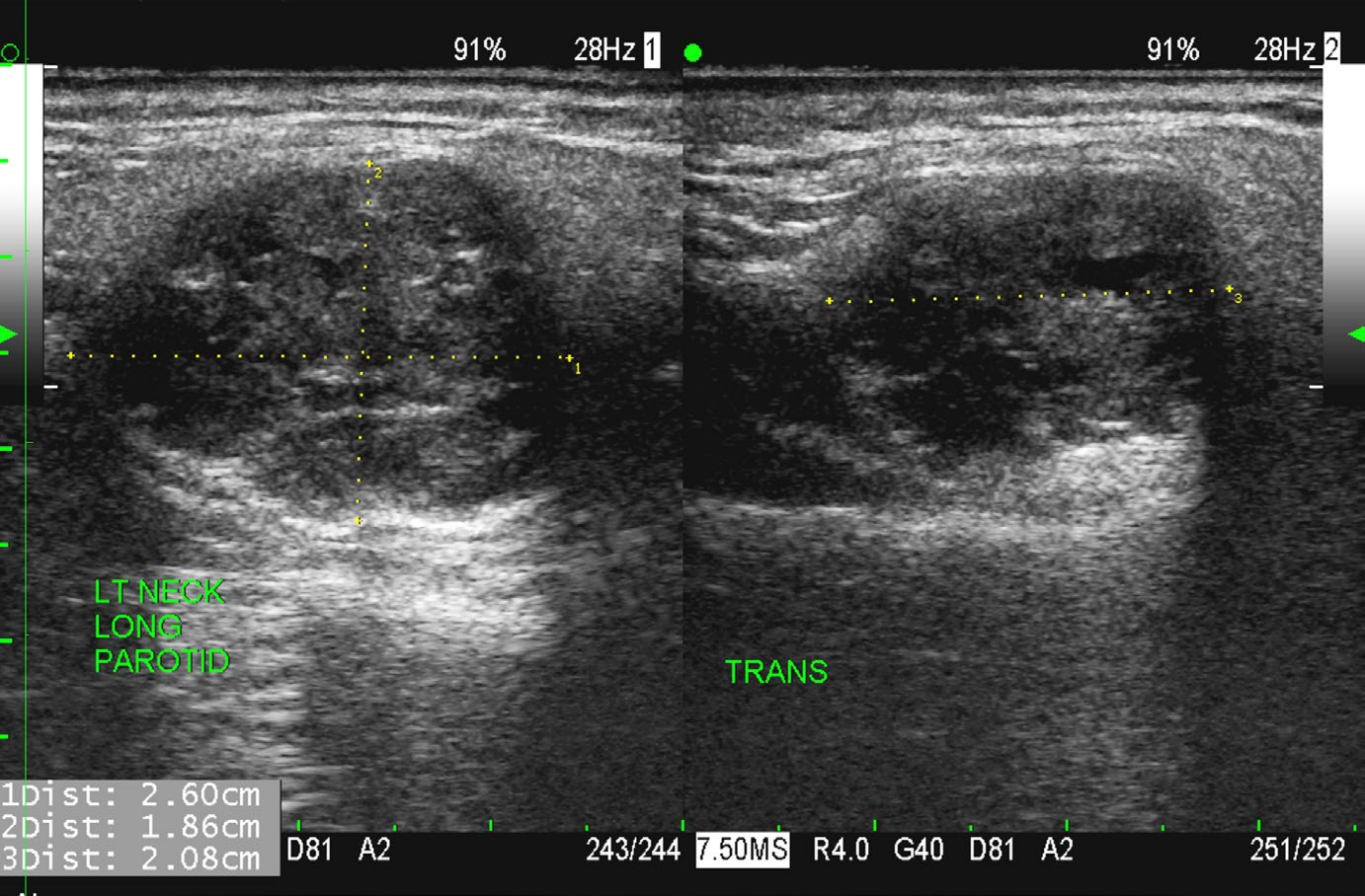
61‐y‐old man with benign mixed tumor (pleomorphic adenoma) of the left parotid gland. Ultrasound of the left parotid lesion reveals a slightly heterogeneous echotexture but a predominantly hypoechoic lesion with well‐defined borders and mild posterior acoustic enhancement. The lesion size measured with ultrasound, 2.6 × 2.1 × 1.9 cm, agreed with the size measured with CT
